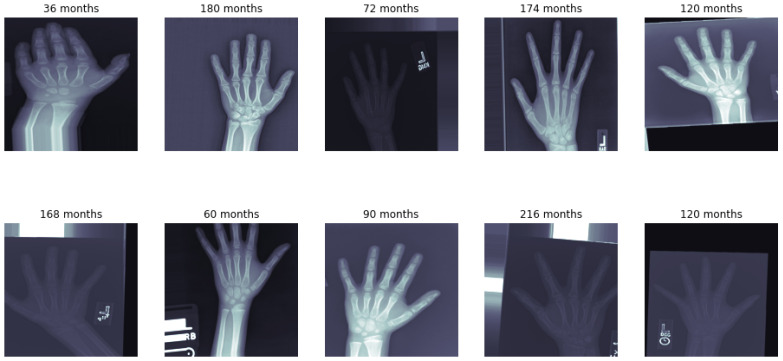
Sample hand and wrist radiograph from the RSNA Bone Age dataset.
